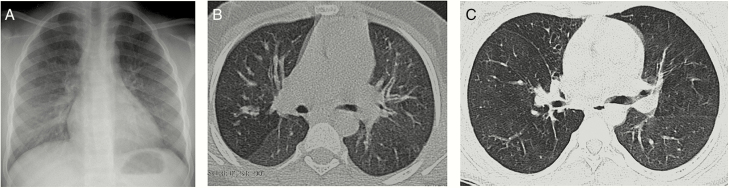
A,* Chest radiograph of a participant scored as normal independently by both radiologists. *B,* High-resolution computed tomographic (HRCT) scan of the same patient, through the midzone demonstrating a striking mosaic pattern with areas of decreased attenuation adjacent to regions of normal lung density. *C,* HRCT scan of another patient, again showing regions of decreased attenuation as part of the mosaic pattern with cylindrical bronchiectasis in the middle lobe.
